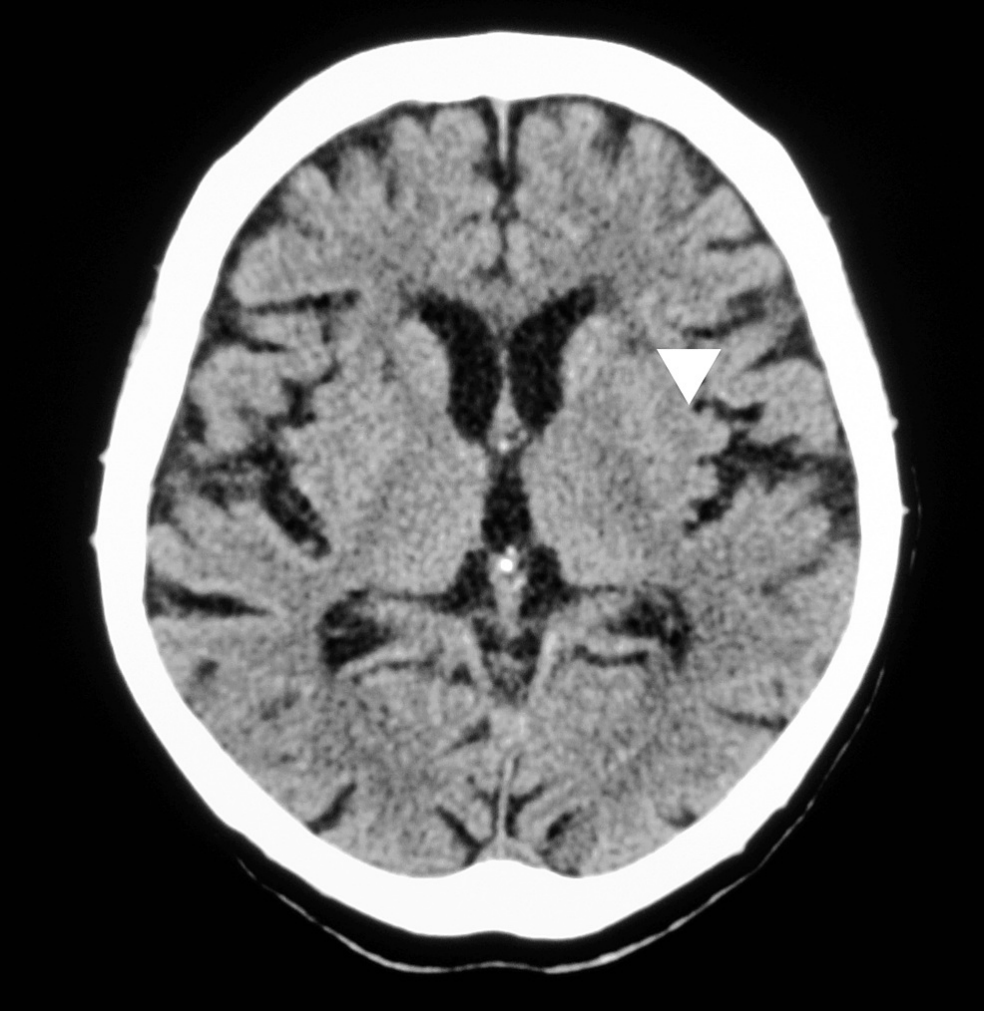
Head CT The white triangle indicated the low-density lesion at the left basal ganglia on head CT.
